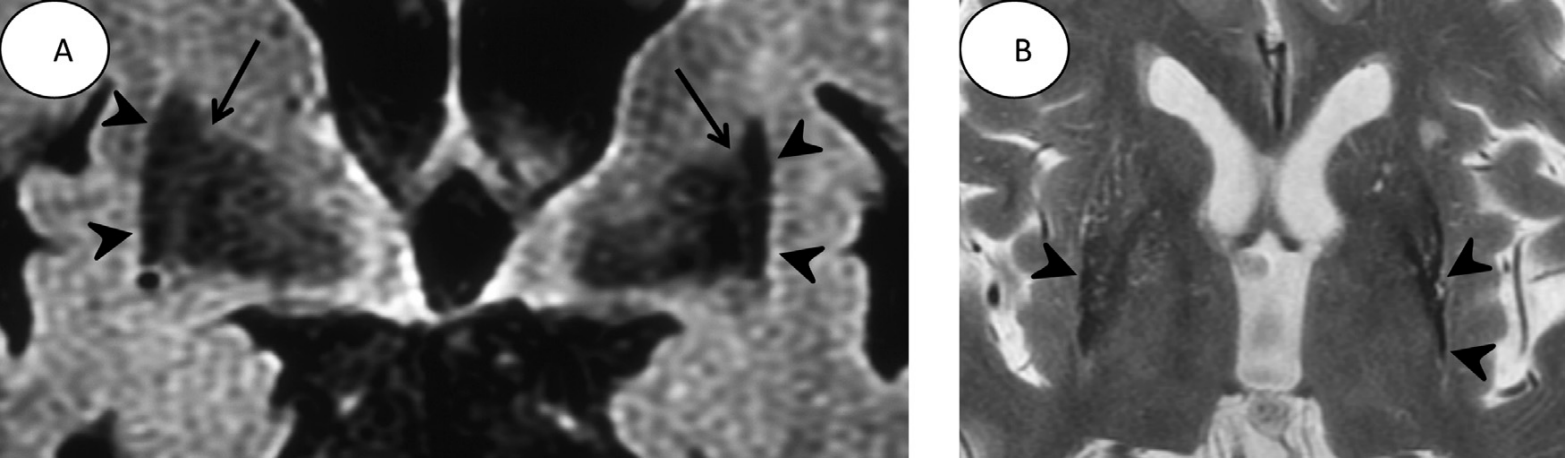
(A) In the coronal FLAIR image taken 2 years later, atrophy is observed in the right putamen (arrowheads) in addition to that in the left putamen (arrowheads). Moreover, the right posterior putamen has hypointensity. The lateral medullary lamine (arrows) can be identified on both sides. (B): T2WI shows progressive atrophy in the left putamen and hypointensity in the lateral side of the putamen (arrowheads). Hypointensity is also observed in the lateral side of the right putamen (arrowhead).
